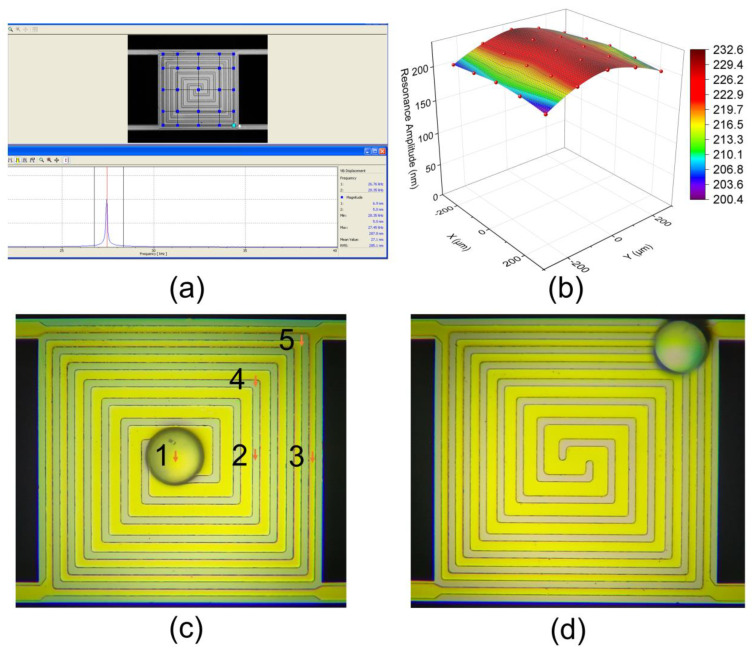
(a) Test interface of amplitude distribution with a laser Doppler analyzer; (b) test results of the amplitude distribution; (c,d) optical images of the resonant beam sample area with PS spheres loaded at different locations.
